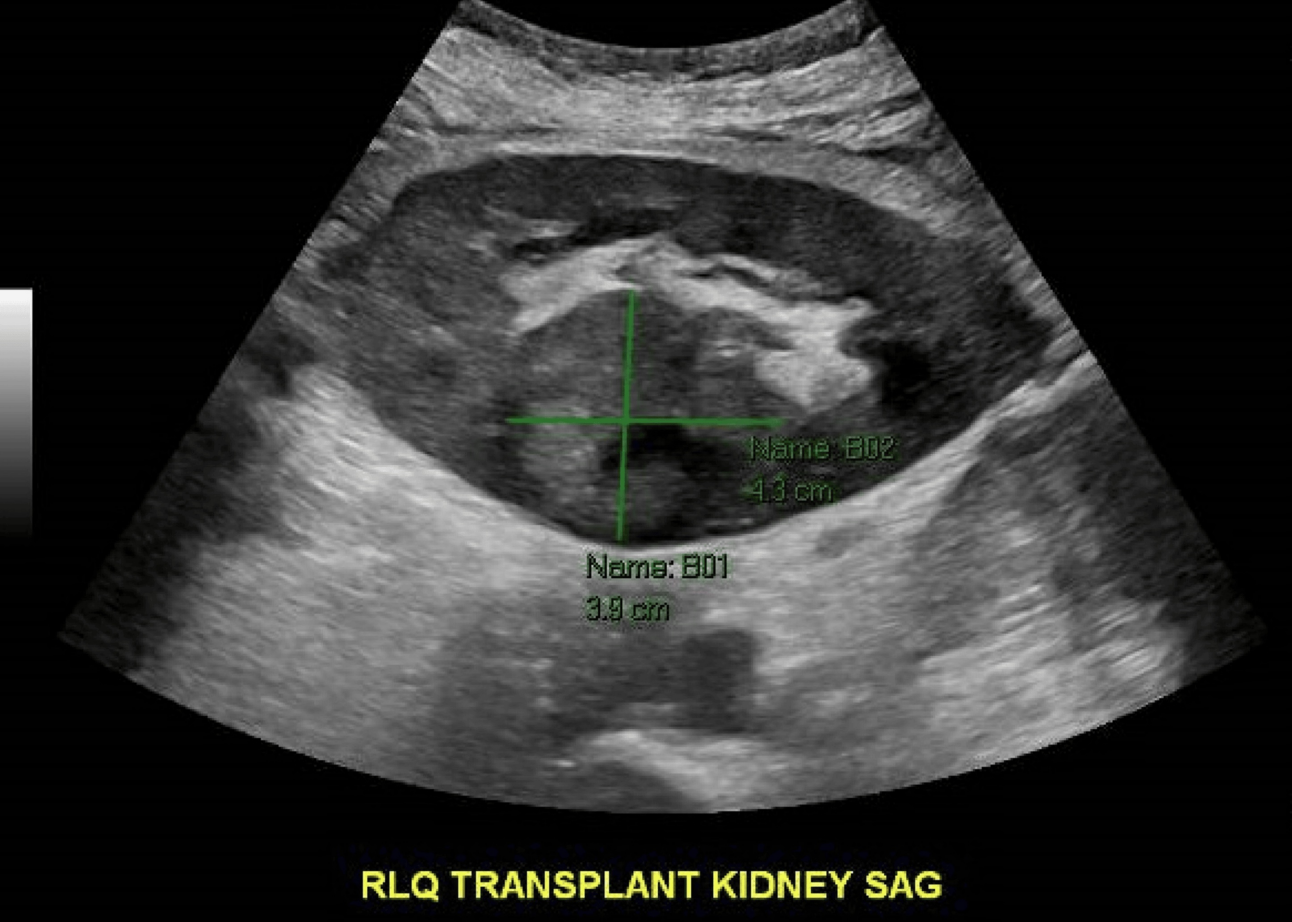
Renal ultrasound The figure depicts a focal rounded contour in the renal cortex which could represent a mass.
